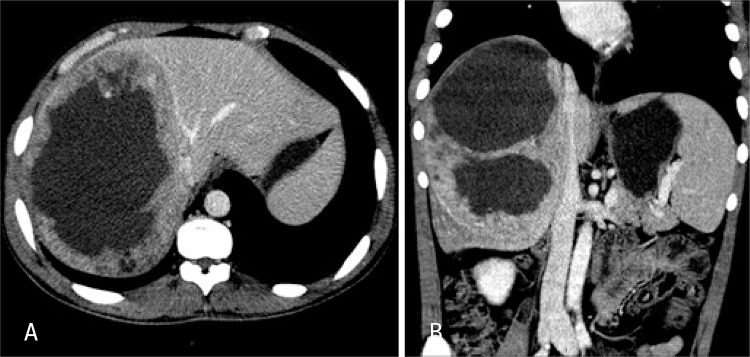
(A) Axial computed tomography image - portal phase - middle hepatic vein - contact with lesion. (B) Coronal computed tomography image
